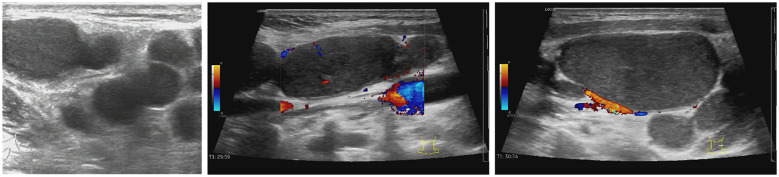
Color Doppler ultrasonography of cervical lymph node biopsy and associated vascular flow.
